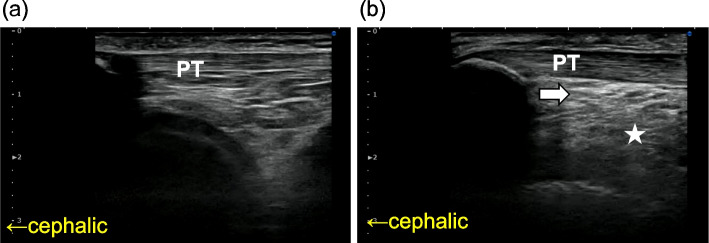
Ultrasound long-axis view of the unaffected (a) and affected (b) knee. White arrow indicates the infrapatellar fat pad with increased brightness; asterisk indicates slow-moving parts of the infrapatellar fat pad in the right knee. PT patella tendon
